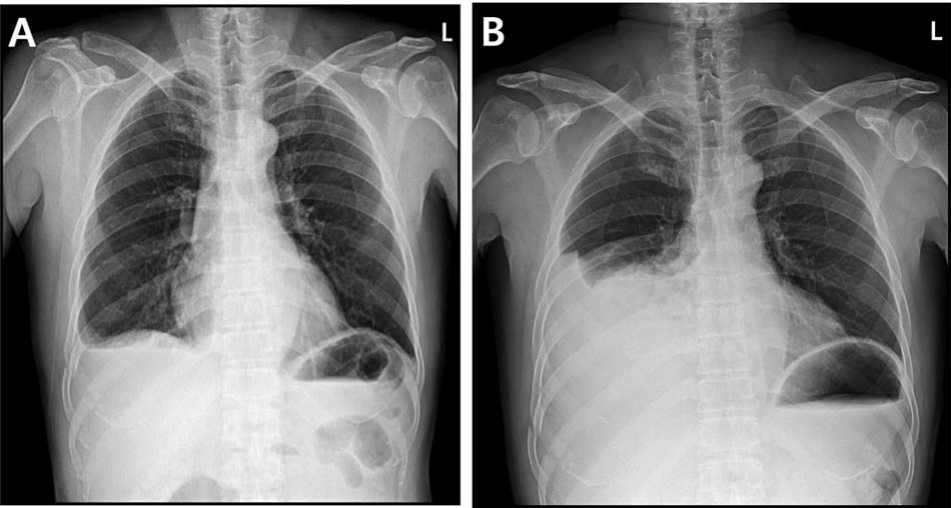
Radiographic findings. (A) Day of symptoms, chest X-ray in the morning. (B) Chest X-ray after symptoms develop.
